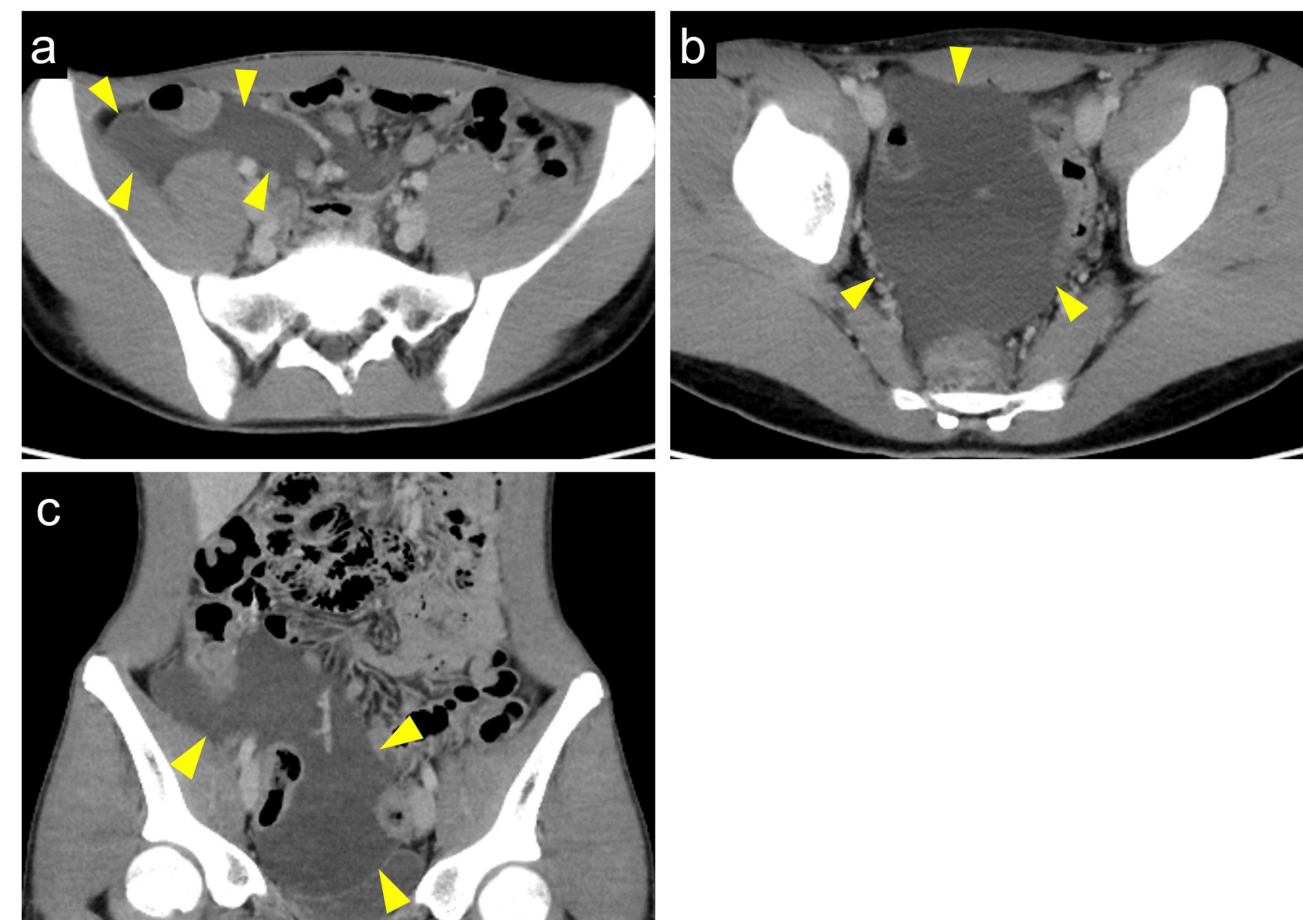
Contract-enhanced computed tomography (CT) showing mesenteric lymphangioma (a) The axial view of CT shows a fluidlike lesion in the right iliac fossa (arrowheads). (b) Another axial view of CT shows a fluidlike lesion in the pelvis (arrowheads). (c) The coronal view of CT shows the fluid-level lesion extending from the right iliac fossa to the pelvis (arrowheads)
